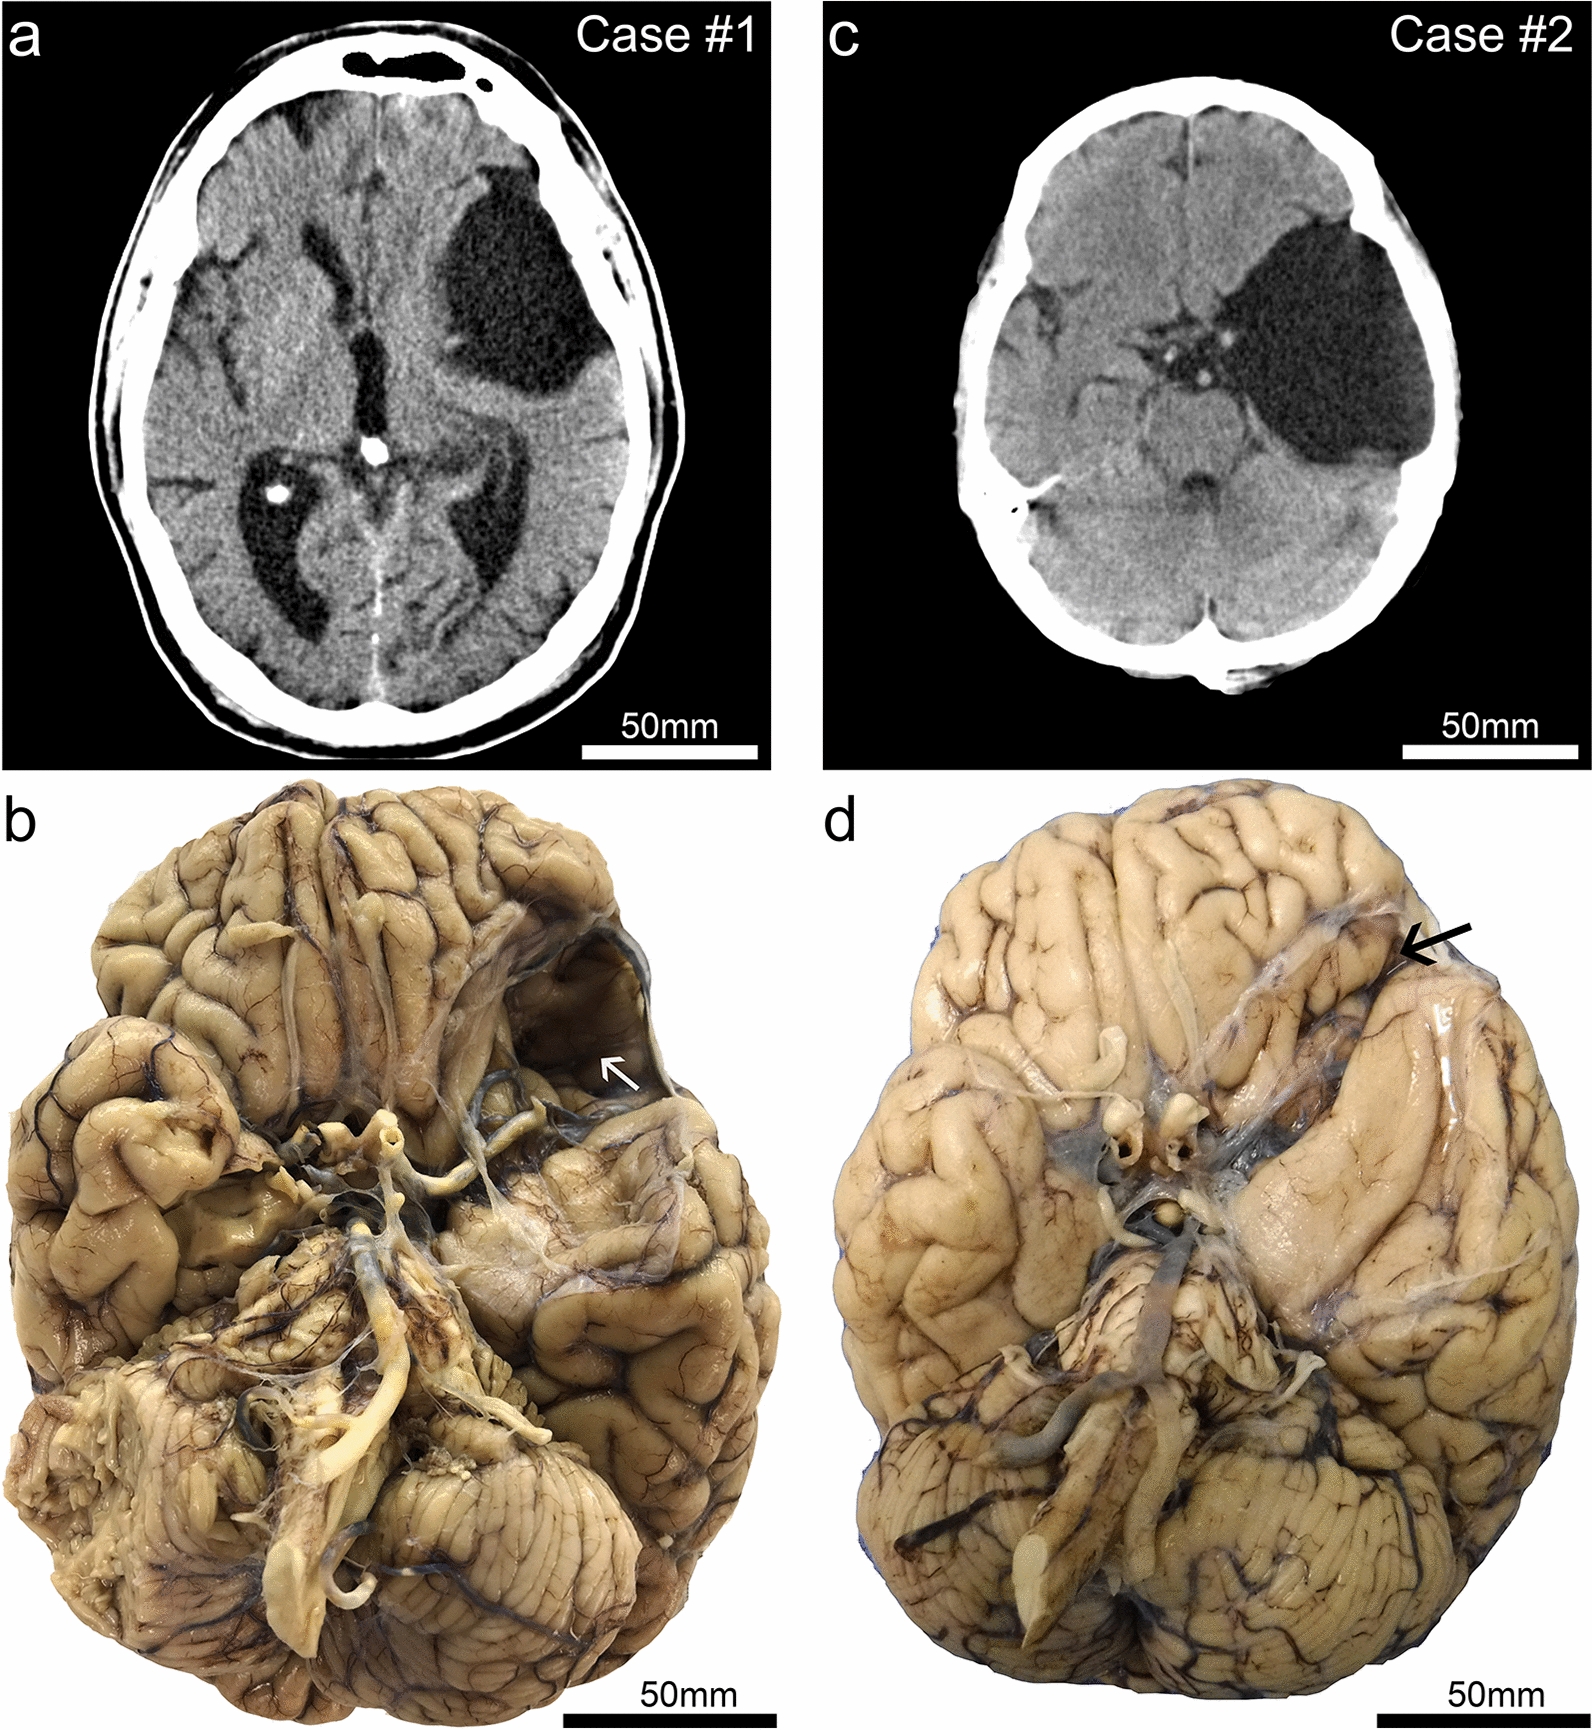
Clinical (premortem) computerized tomography (CT) scans, and photographs of brain after autopsy and fixation, in two cases with large arachnoid cysts. Case #1 is a male, 72 years old at death. This CT scan (a) was acquired at age 68. (b) The brain at autopsy following fixation from Case #1 shows the large left-sided arachnoid cyst cavity. Both the anterior temporal lobe and the orbitofrontal frontal lobe are affected by the lesion. Note that on the surface of the frontal cortex within the cyst cavity there is discoloration (white arrow). By contrast, the right side lacks evidence of indentation or contusion in the orbitofrontal cortex. Case #2 is a male, 64 years old at death. The CT scan (c) was acquired at age 56. As seen in the brain at autopsy following fixation (d), the arachnoid cyst is larger in the rostral-caudal axis in Case #2 in comparison to that of Case #1. However, the lesion is shallower—the affected region is smaller in the dorsal–ventral axis, and there was less impact on the orbitofrontal cortex (black arrow) in Case #2 compared to Case #1
